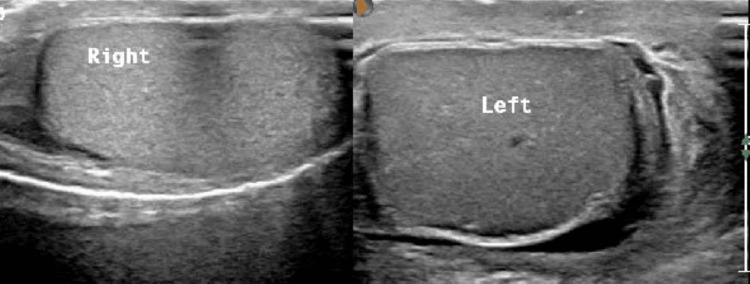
Scrotal ultrasound scan showing hypoechoic left testicle
